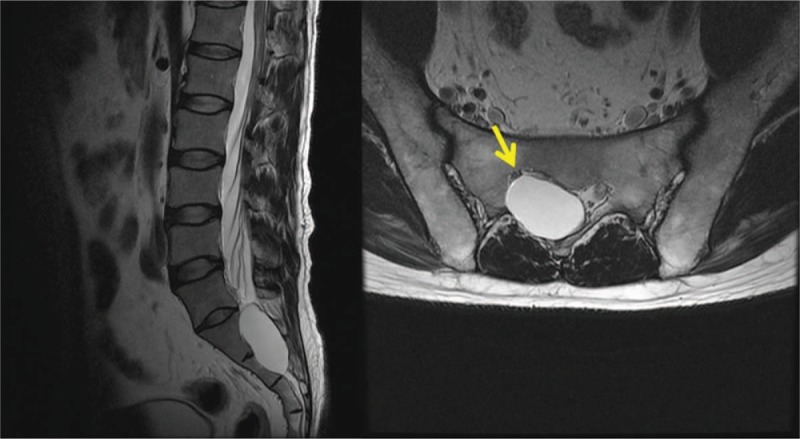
Magnetic resonance imaging of the patient complaining of low back pain and right posterior thigh pain. A large perineural cyst is observed (size: 53 × 31 × 21 mm). The arrow indicates the compressed right S1 nerve root.
